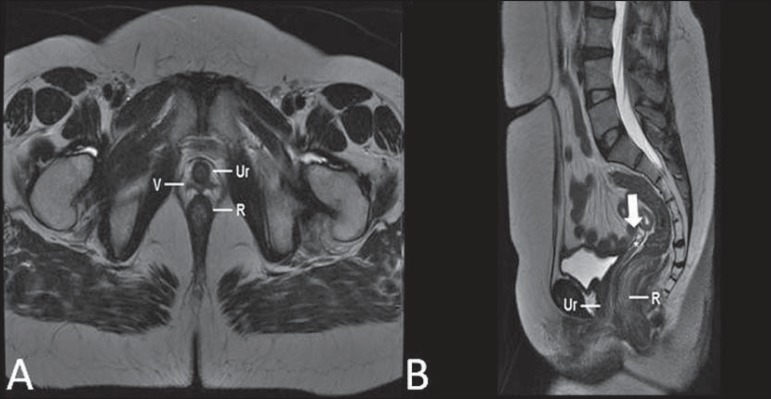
Turner syndrome. Multiplanar MRI T2-weighted sequence (A,B)
